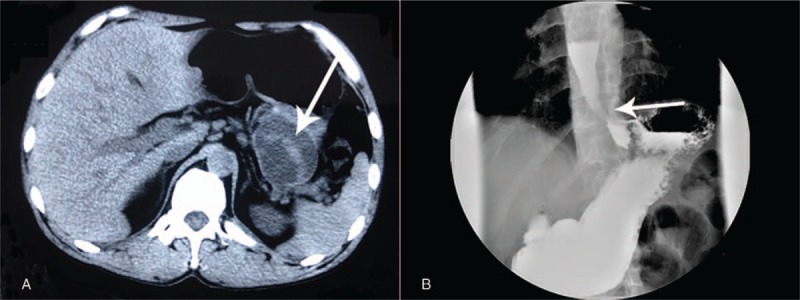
Diagnostic computed tomography (CT) and upper gastrointestinal radiography. (A) CT obviously enlarged cystic mass of pancreas, (B) Esophageal stenosis (white arrowhead).
